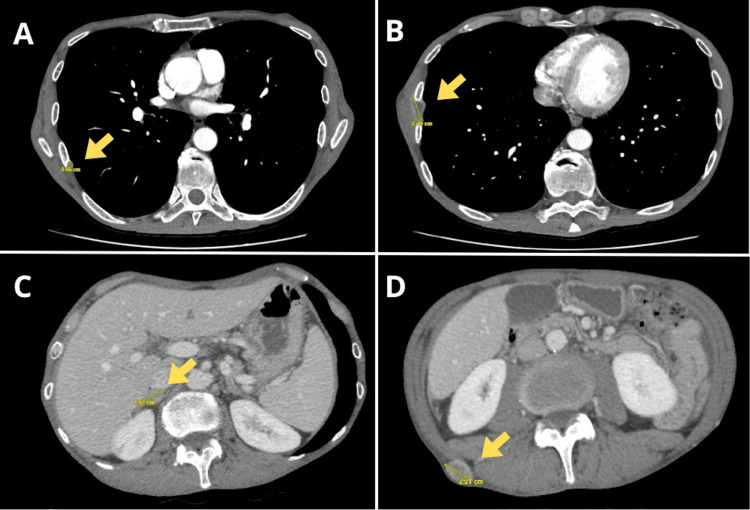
Thoracoabdominal CT, November 2020. Pleural (A, B), adrenal (C), and subcutaneous metastases (D) are observed.
